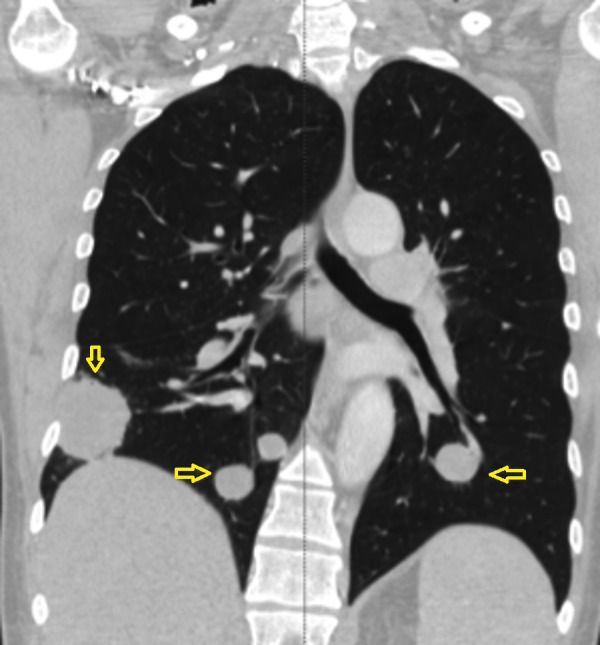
Coronal reconstructive CT scan of the chest showing multiple masses in the lung (arrows). CT - computed tomography.
